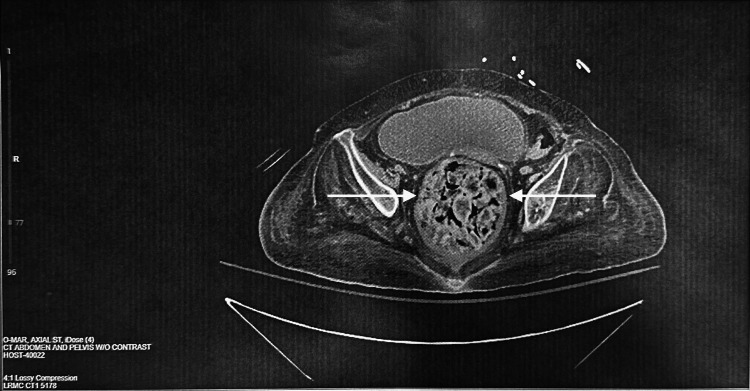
CT abdomen and pelvis w/o contrast Fecal impaction in the rectum with mild wall thickening and surrounding fat stranding, suggestive of stercoral colitis/proctitis, is seen. The moderate to large colonic stool burden is suggestive of constipation.
